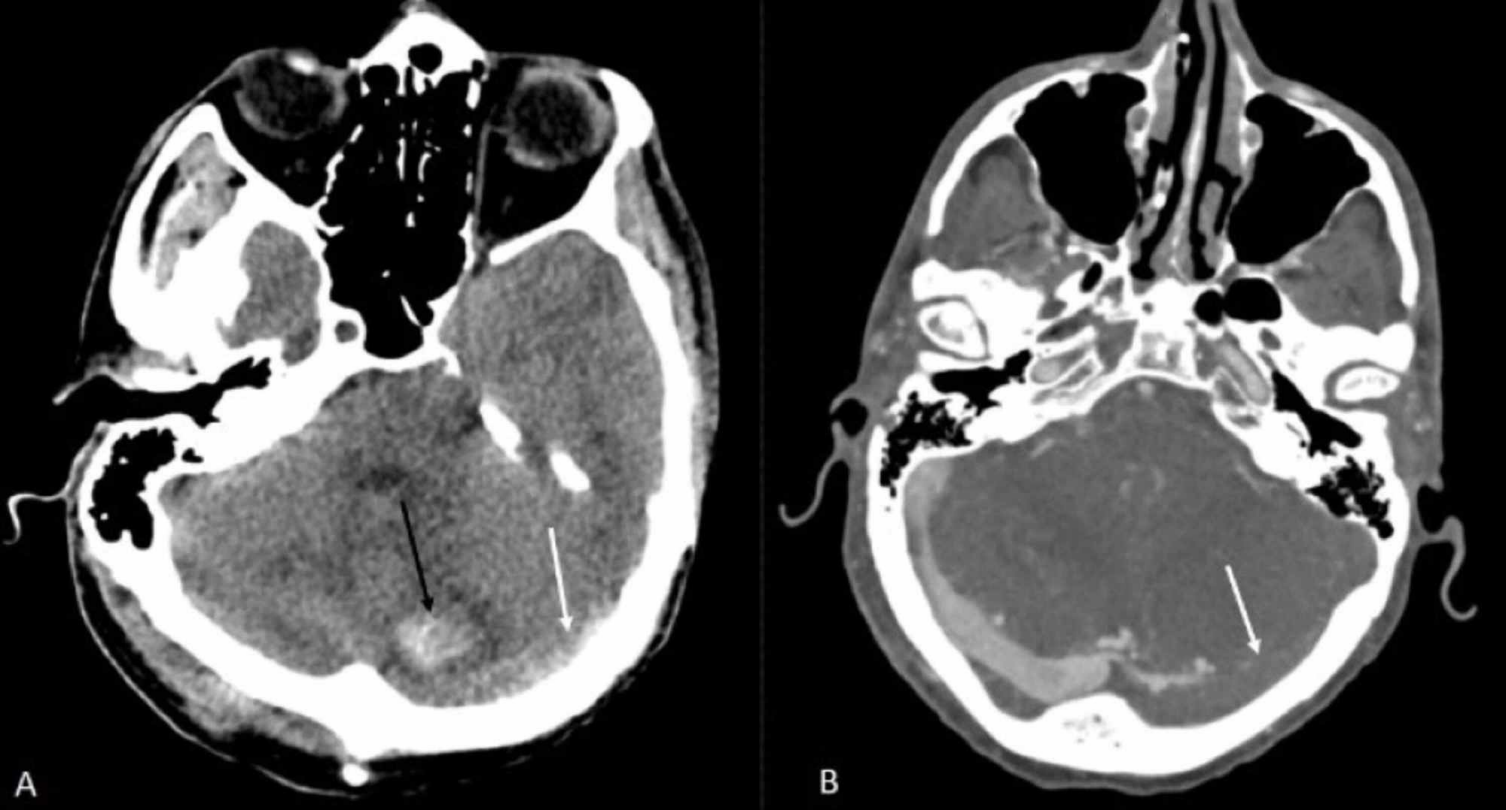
Axial non-contrast CT and CT venogram of head (A) Axial non-contrast CT head showing left cerebellar hemorrhage (black arrow) and a dense left sigmoid sinus consistent with sigmoid thrombosis (white arrow), (B) Axial CT venogram of the head showing non-opacification of the left sigmoid sinus (white arrow) and well opacified right sigmoid sinus.
